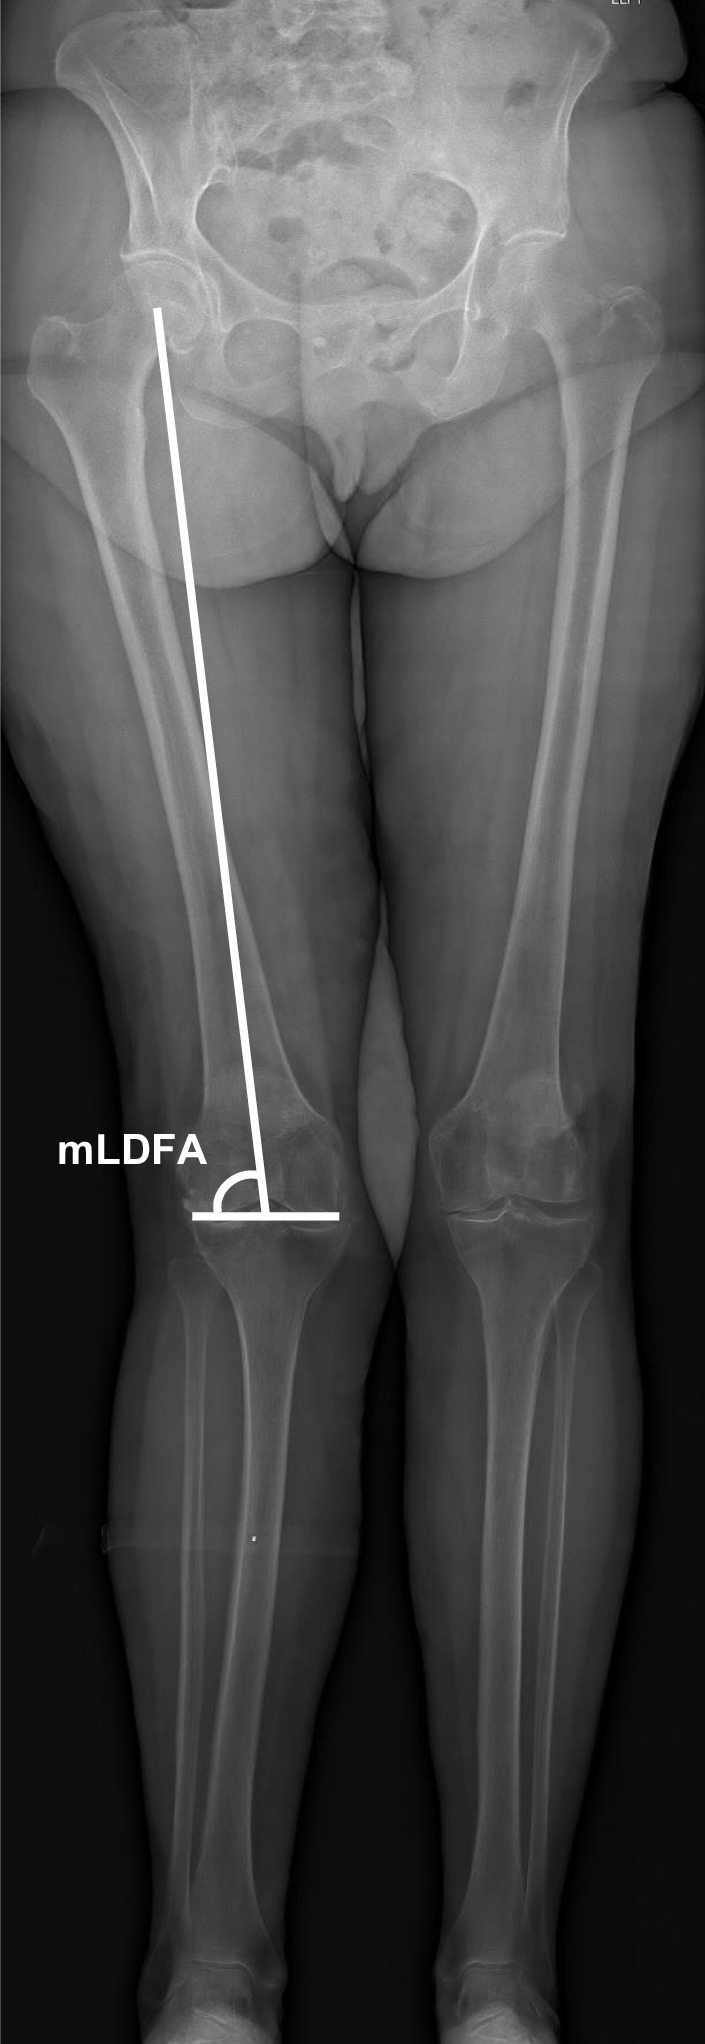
Mechanical lateral distal femoral angle (mLDFA). A pre-operative weightbearing lower-limb anteroposterior EOS digital radiograph is shown. The mLDFA is the angle between the mechanical axis of the femur and its articular surface
